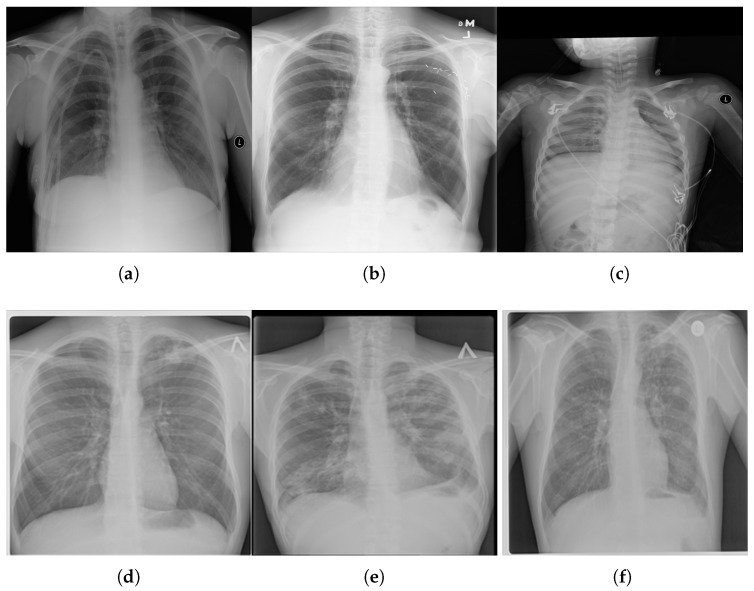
Examples of chest X-ray images: normal (top row) and tuberculosis (bottom row). (a) Normal 1; (b) Normal 2; (c) Normal 3; (d) Tuberculosis 1; (e) Tuberculosis 2; (f) Tuberculosis 3.
